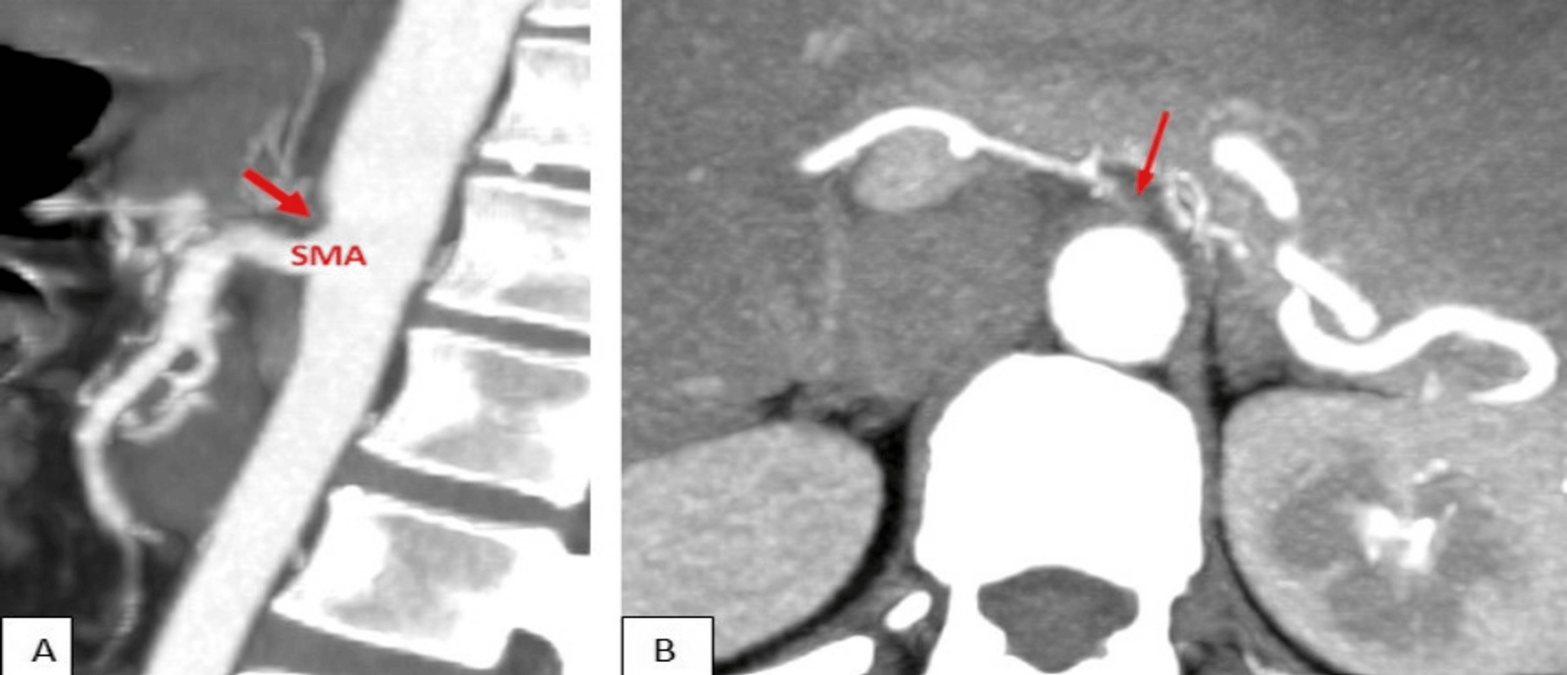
CECT abdomen, sagittal (A) and axial (B) views, of a 40-year-old woman with pain in abdomen (Case 1) Maximum intensity projection (MIP) images of the arterial phase show complete non-visualization of the celiac trunk with several small arterial collaterals in the region where the celiac artery distribution was expected CECT: contrast-enhanced computed tomography; SMA: superior mesenteric artery
